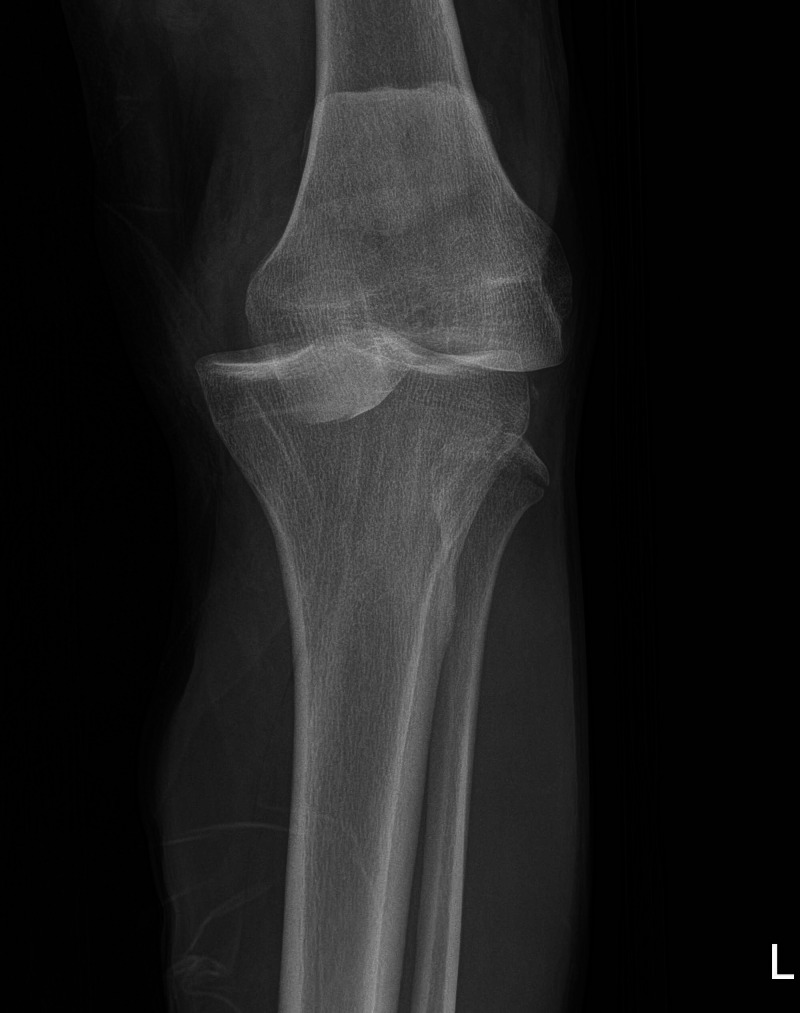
Anterior–posterior radiograph of the left knee in patient 2 Anterior–posterior radiograph of the left knee demonstrating acute knee dislocation.
